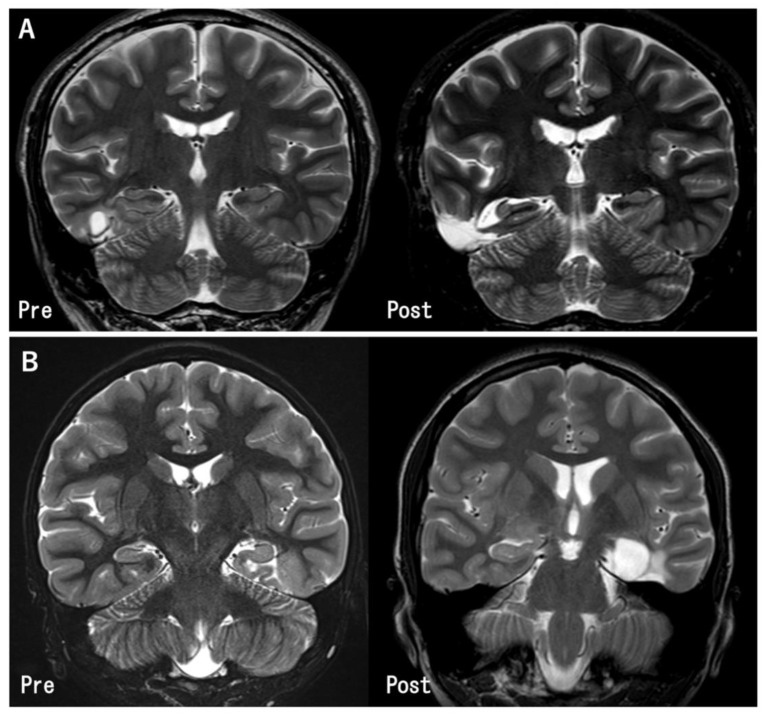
Pre- and post-operative MR images in the representative cases. (A) The tumor in the inferior temporal gyrus was totally removed. The hippocampus was preserved because it was located apart from the tumor (Case 11). (B) The tumor was located at the left parahippocampal gyrus. Gross total tumor removal and hippocampectomy was performed (Case 27).
